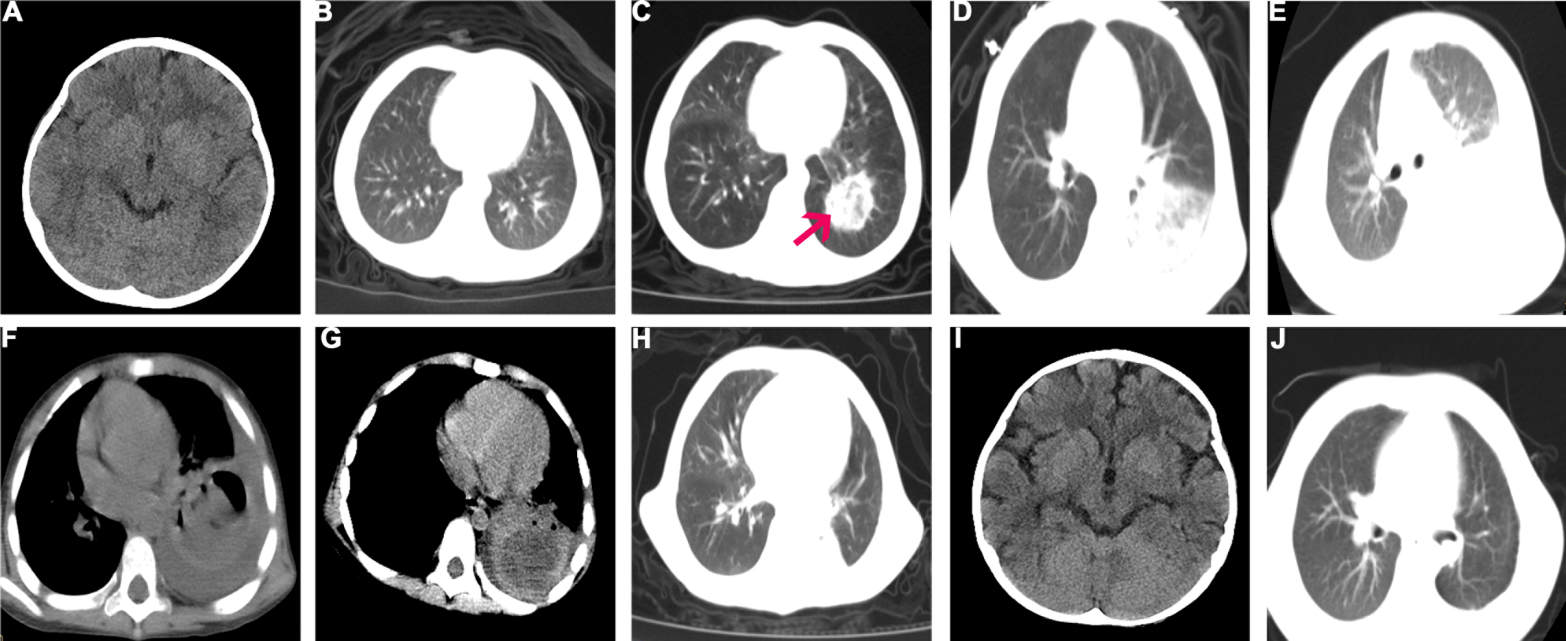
Brain and Chest CT before and after surgery. (A) Brain CT before chemotherapy; (B) Chest CT before chemotherapy; (C) Day 30, a nodular lesion (3.2 cm × 2.8 cm, red arrow) in the post-basal segment of the left lower lobe with ground glass opacification; (D) Day 38, the initial nodular lesion was larger, and new lesion in the upper lobe of the right lung; (E, F). Day 52, the initial nodular lesion was 4.7 cm × 24.3 cm with part consolidation and the balloon cavity; the initial regional hazy shadow was spread diffusely in the left lung. (G) No regression of lesions and fungal flora in blood vessel on CTA (I) Brain CT on 6 months after surgery; (J) Chest CT on 6 months after lung lobectomy, no progression of mucormycosis.
